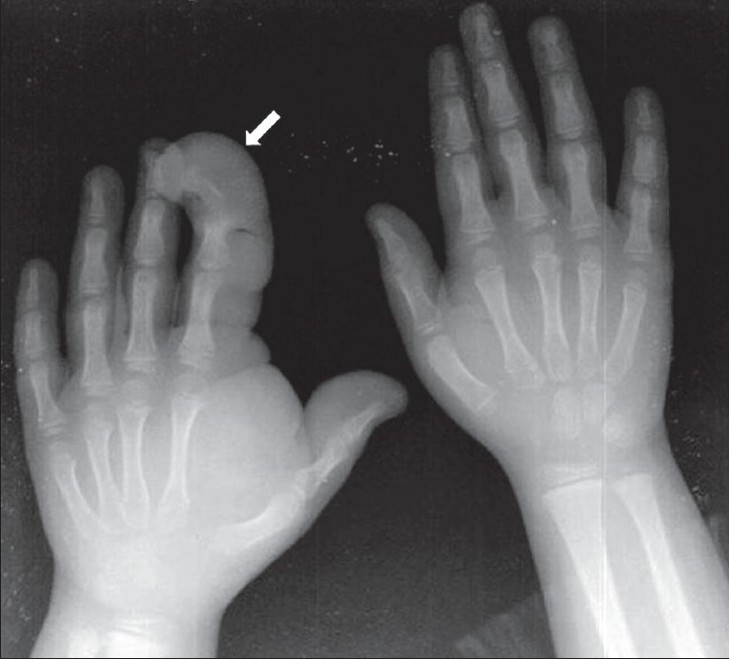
Case 1: Plain radiograph of both hands shows soft tissue swelling (arrow) on the palmar aspect of the affected fingers; the distal end is predominantly affected, with splaying of the phalanges
